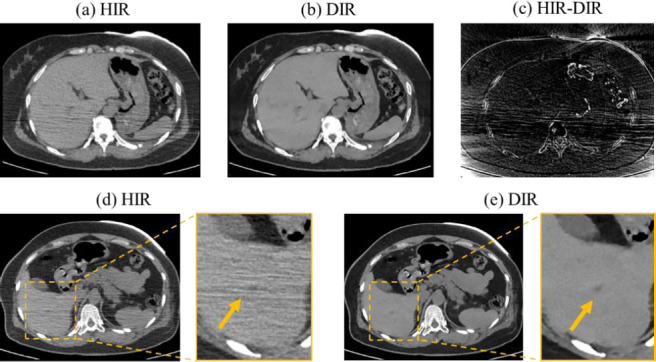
Non-contrast abdominal CT obtained in a 53-year-old woman with irregular arm positioning. Images were acquired at 9.322 mGy and reconstructed with HIR and DIR. At the level with the most severe artifacts (a~c), image noise and streak artifacts are reduced by DIR. Subtraction images of HIR-DIR (c) reveal no loss of anatomical details aside from bone structures, indicating that anatomical detail has been well preserved. At another axial level (d, e), the hypoattenuating liver cyst is obscured by the severe streak artifacts on the HIR images but sharply delineated on the DIR image.
